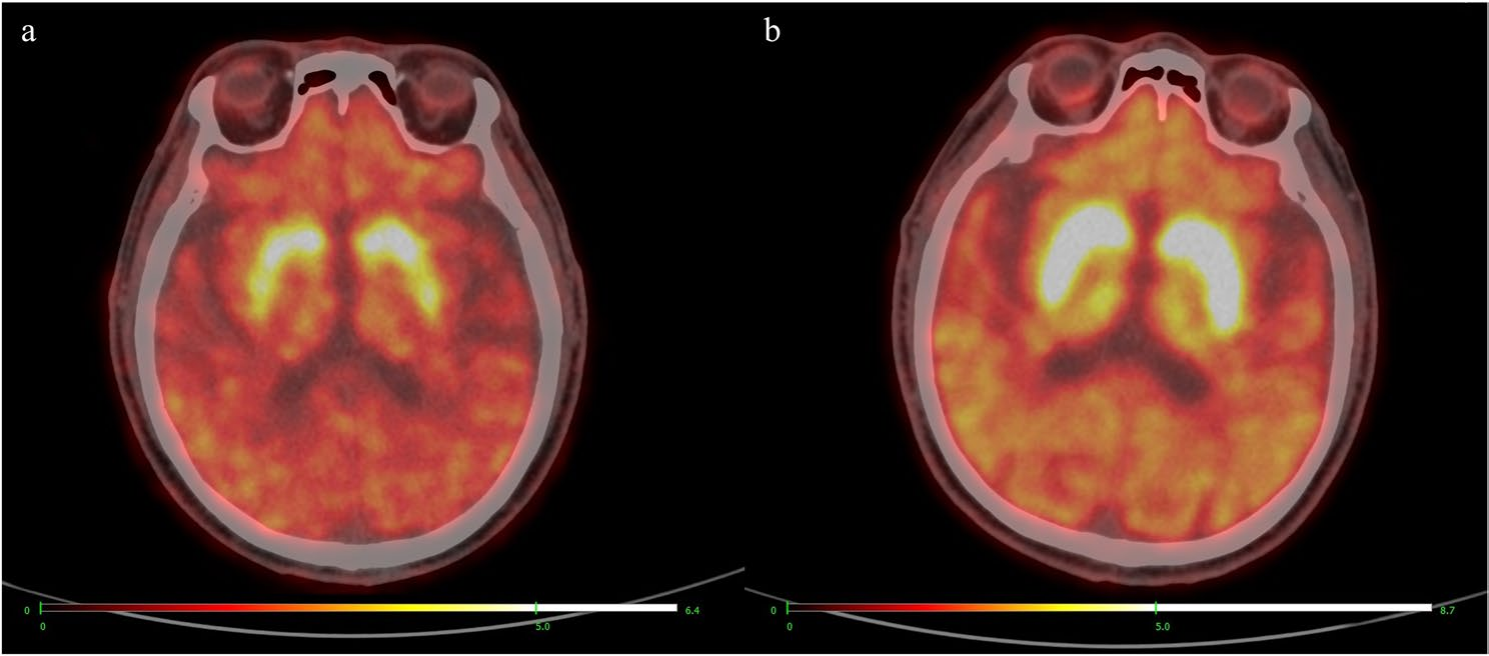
Typical striatum ^11^C-CFT PET/CT imaging. a. PET/CT fusion images of PD patients; b. PET/CT fusion images of healthy controls. Images are displayed on an identical fixed SUVmax scale for accurate visual comparison
